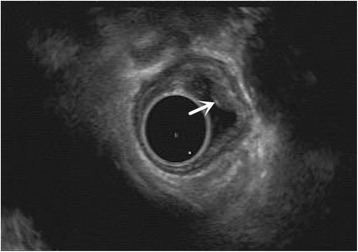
Endoscopic ultrasound examination shows an intramural subepithelial nodule
